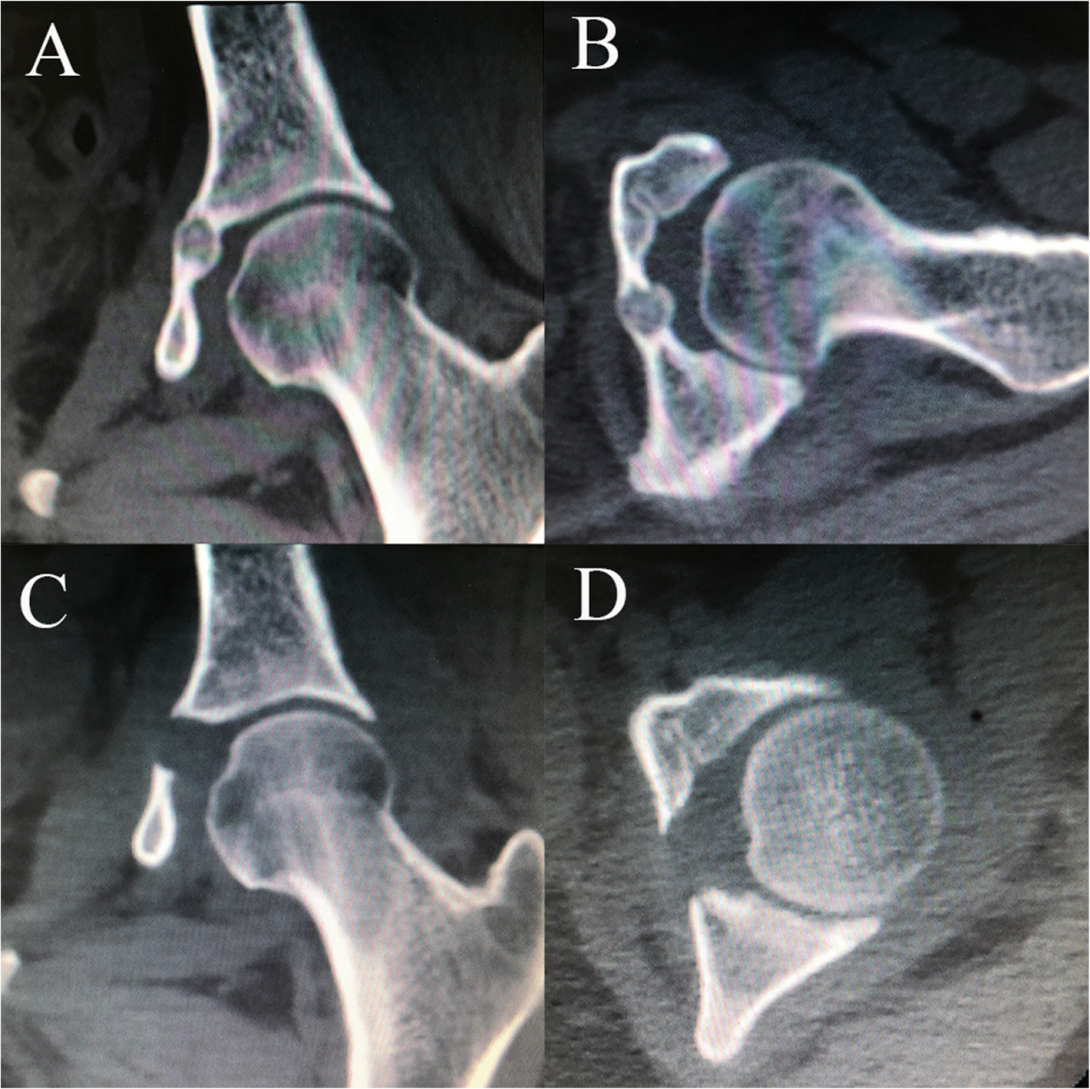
Preoperative and postoperative CT of one patient diagnosed with OO of the acetabulum in zone 6. A, C. Preoperative and postoperative coronal CT. B, D. Preoperative and postoperative axial CT
